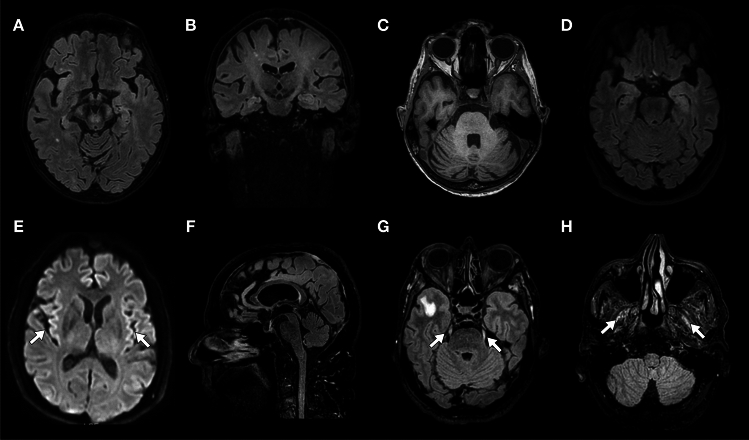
Brain magnetic resonance imaging findings. Mesencephalon (A) and pyramidal tracts (A, B) T2/fluid attenuated inversion recovery (FLAIR) hyperintensity, and pontocerebellar atrophy (C; case n. 47). Left medial temporal lobe T2/FLAIR hyperintensity (D; case n. 16). Diffusion-weighted imaging cortical hyperintensity (E, arrows; case n. 6). Mesencephalic atrophy (F; case n. 5). T2/FLAIR hyperintensity of the trigeminal nerves (G, arrows) and lateral pterygoid muscles (H, arrows; case n. 4)
